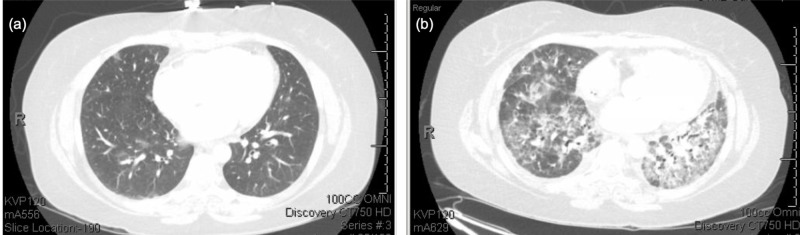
High-resolution computed tomography of the chest for patient 2. (a) High-resolution computed tomography of the chest for patient 2 prior to initiation of chemotherapy, (b) High-resolution computed tomography of the chest for patient 2 after three cycles of mFOLFOX + bevacizumab showing extensive bilateral ground-glass opacities.
